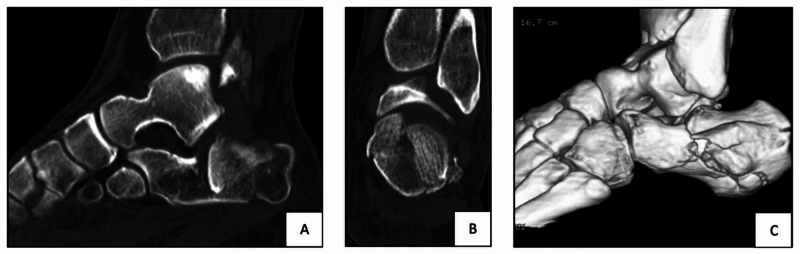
Sagittal (A), coronal (B) and three-dimensional reconstruction (C) CT scan of the calcaneal fracture treated by MIS fixation. *Same patient’s intra-operative photograph and fluoroscopic images are shown in Figures 2, 3, respectively. MIS, minimally invasive surgery
